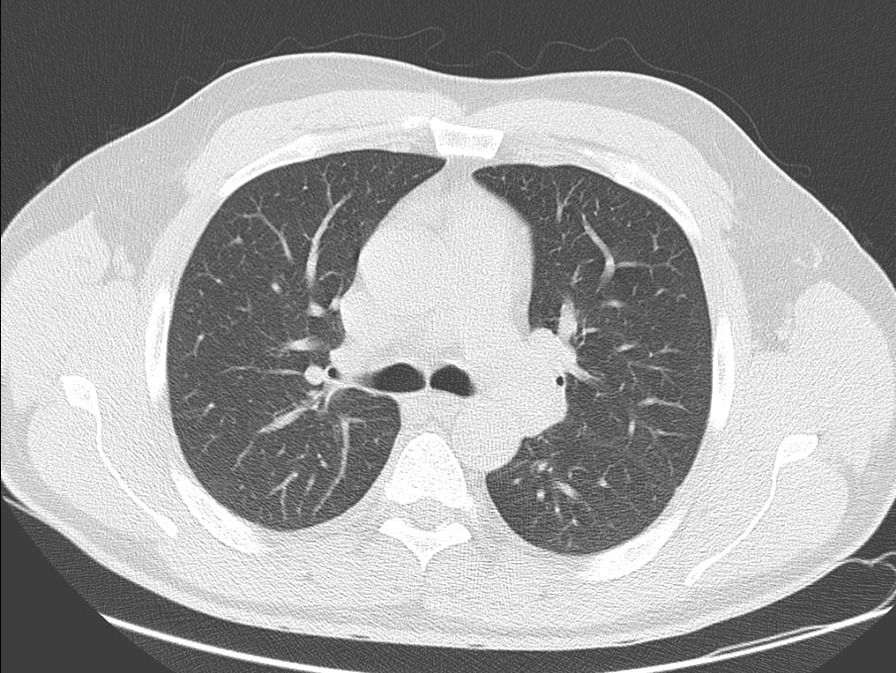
Showing the patient thorax CT on the day of hospitalization. CT revealed the patient's thorax was not involved by the disease
